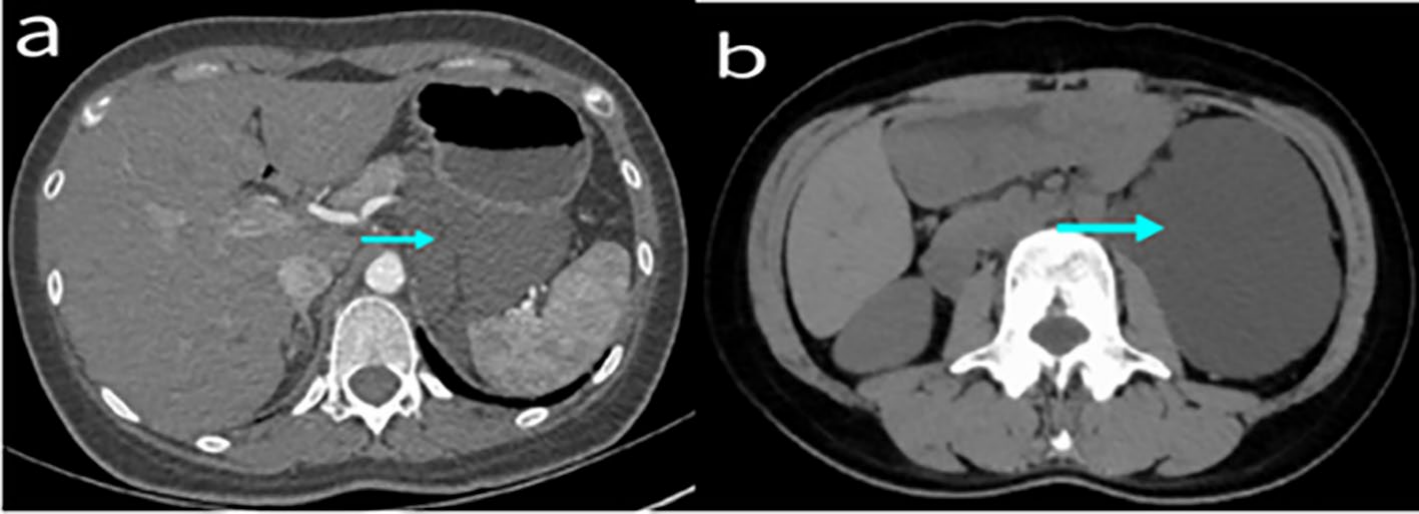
Figure a shows Enhanced CT images of retroperitoneal lymphangioma; Figure b shows CT scan without contrast
